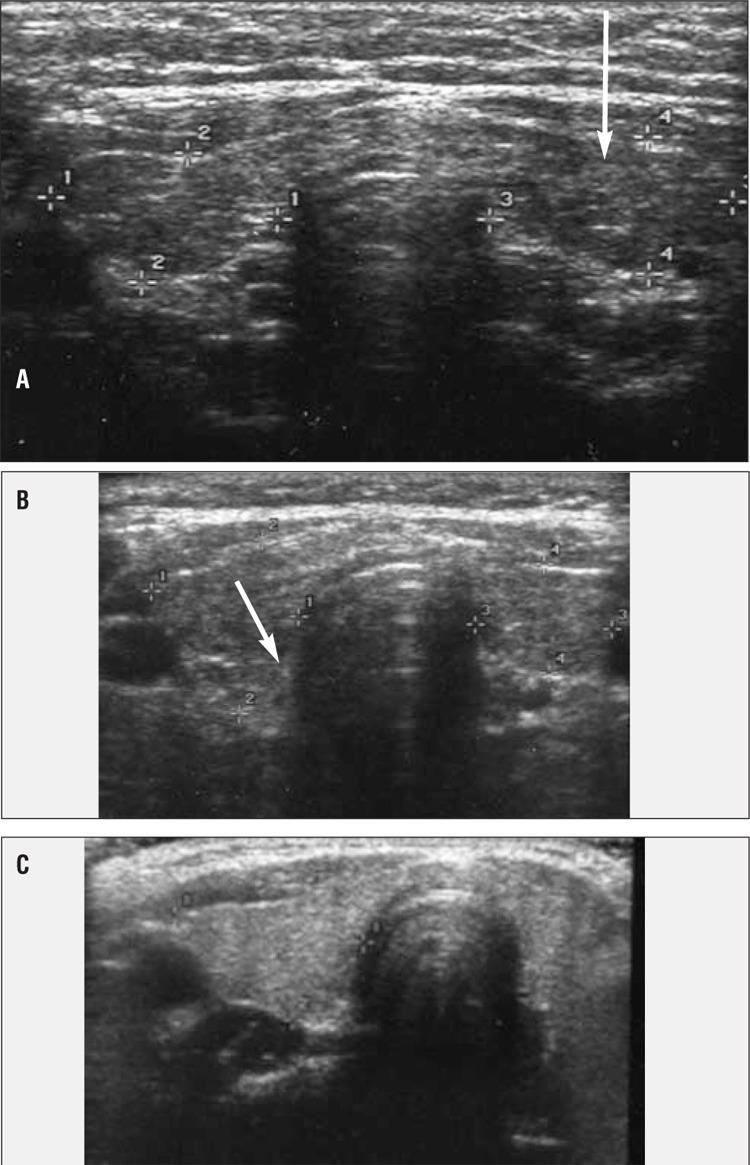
Thyroid ultrasound images in a subject with Hashimotothyroiditis (A), in an obese child (B) and in a normal subject (C)
